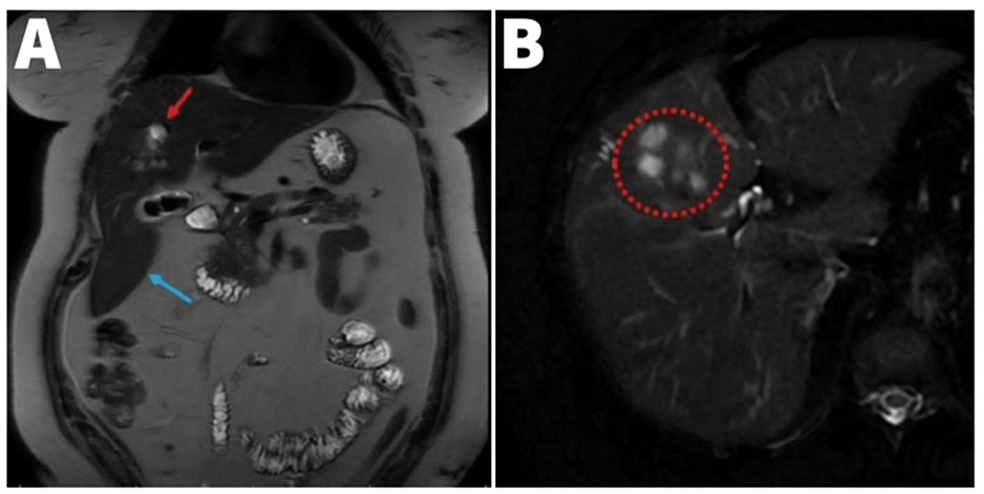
MRI of the abdomen and pelvis. MRI of the abdomen and pelvis (A) showing a complex appearing lobular mass (red arrow) measuring approximately 4.2 x 5.0 x 4.3 cm at the junction of the left and right lobe (segment IV to segment VIII) and elongated tongue-like extension of the right lobe of the liver inferiorly consistent with the Riedel's lobe (blue arrow) and (B) enlarged view of liver showing the complex appearing lobular mass (red dotted circle). MRI: magnetic resonance imaging.
